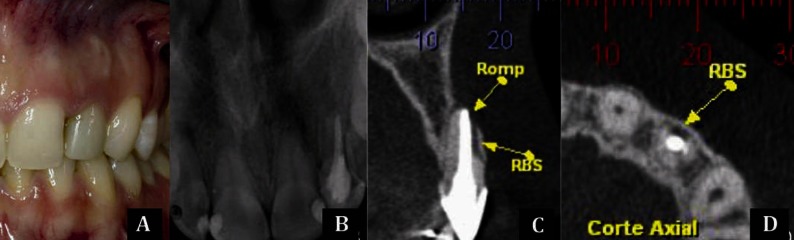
A) Clinical aspect of tooth 22; B) Periapical radiograph of anterior teeth showing no evidence of root fracture; C,D) CBCT images in the sagital and axial planes of the tooth showing an evident root fracture (FR) with separation of the fragment
